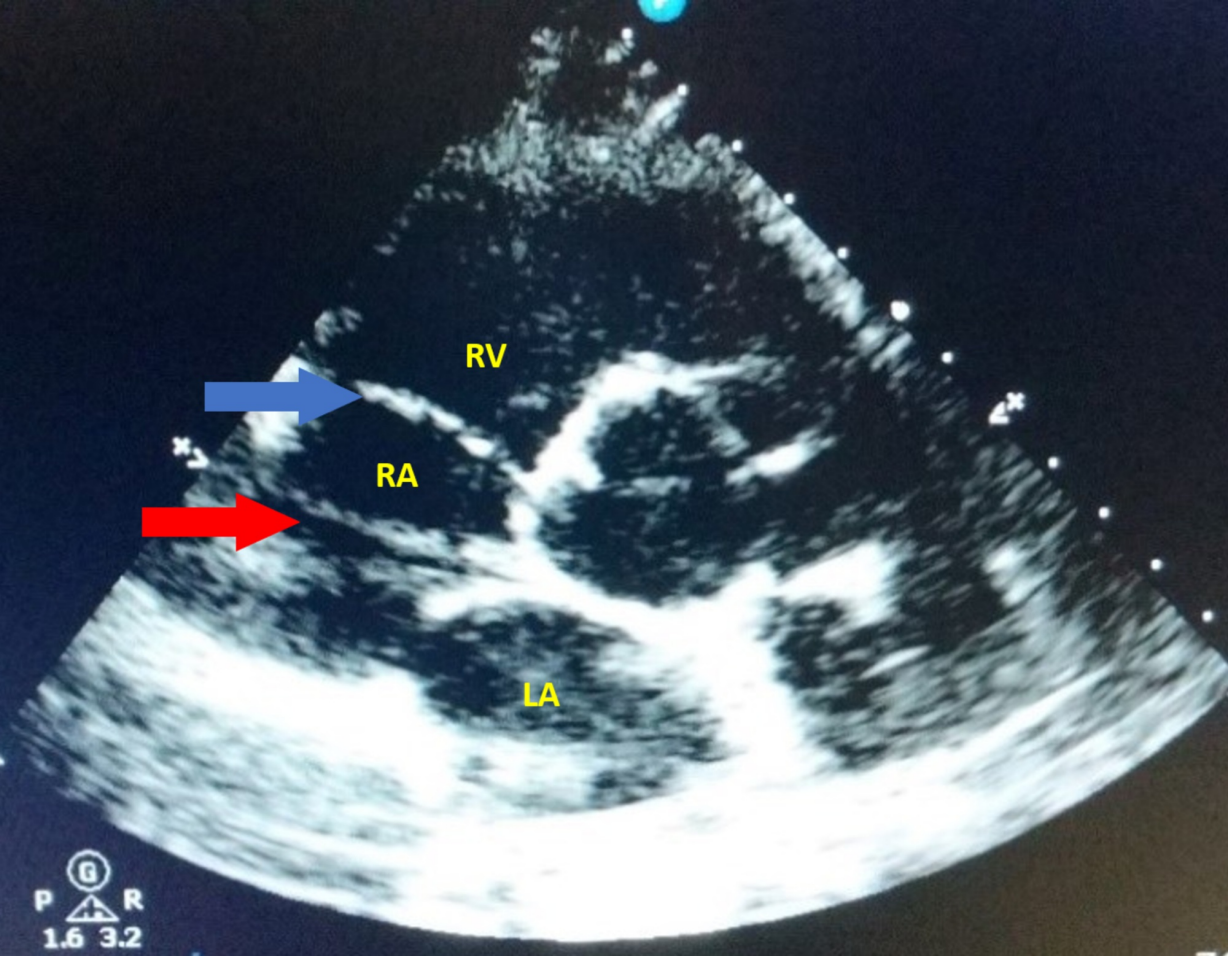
Transthoracic echocardiogram (TTE). Parasternal short-axis view showing a membranous structure (CTD) within the right atrial cavity (red arrow) and tricuspid valve (blue arrow). Abbreviations: LA, left atrium; RA, right atrium; RV, right ventricle
